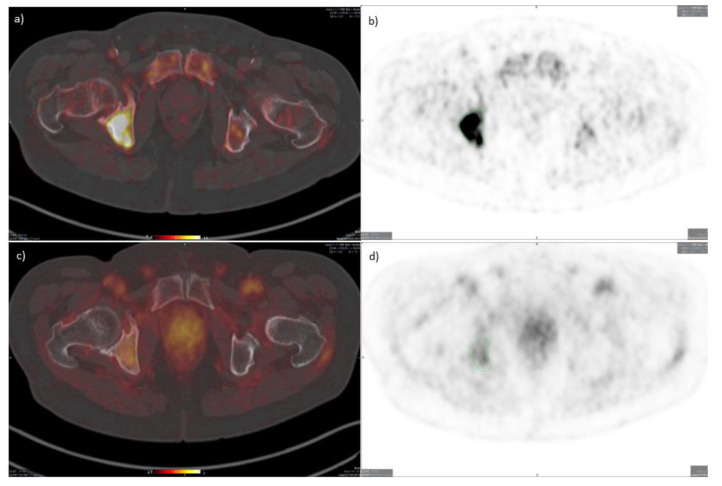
^18^F-fluoride and uPAR-PET/CT (patient 3) Transaxial fused images (left), PET images (right). (a,b): ^18^F-fluoride PET/CT. (c,d): uPAR-PET/CT (bottom row). Bone metastasis in the pelvis is delineated. uPAR PET SUVmax = 2.9.
